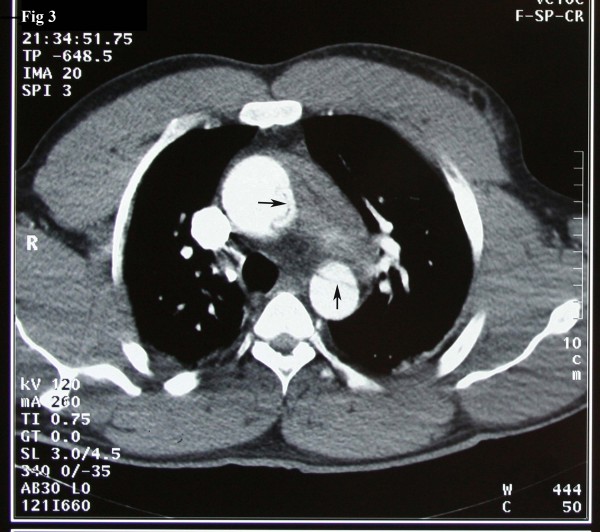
Axial source image of CT angiography reveals the flap in the descending aorta compatible with type A of Stanford dissection: Arrows are showing the flap in descending aorta.
